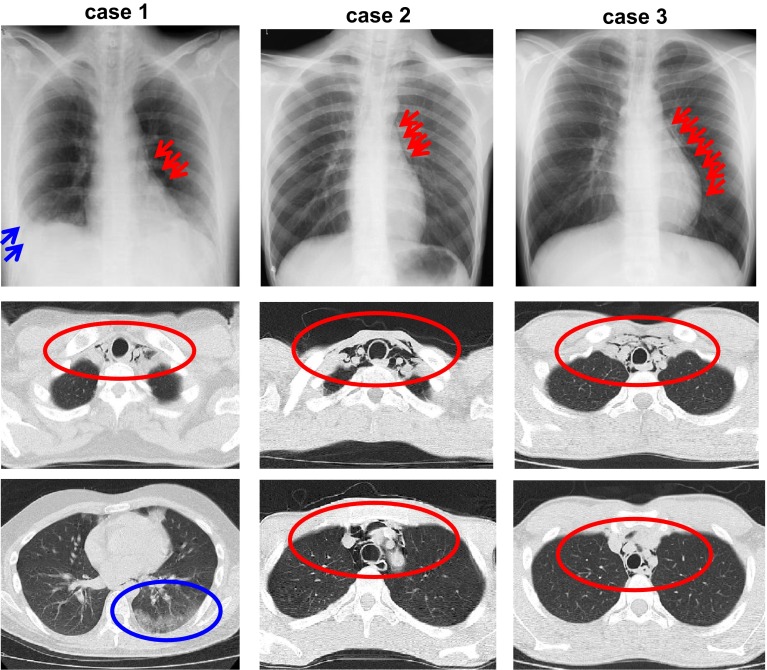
Chest X-ray (*upper panels*) and computed tomography (*middle* and *lower panels*) in three cases with Hamman’s syndrome triggered by the onset of type 1 diabetes mellitus accompanied by diabetic ketoacidosis. Pneumomediastinum is observed in these three cases (*red arrows* and *red circles*). In case 1, pneumonia image is observed in lower right lung field (*blue arrows* and *blue circle*)
